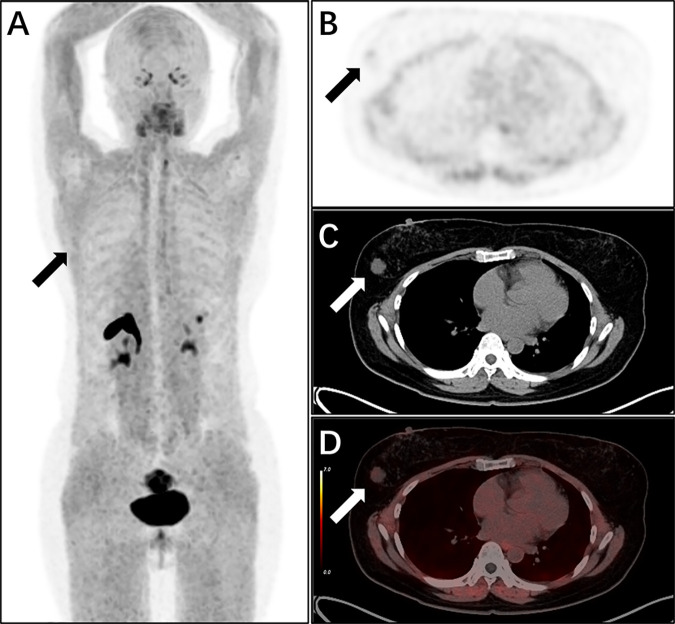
Al¹⁸F-NOTA-FAPI-04 PET/CT images of a 50-year-old female patient with breast mucinous carcinoma (molecular subtype: HER2-positive). (A) Whole-body maximum-intensity projection (MIP) image reveals a mildly FAPI-avid lesion (low to moderate tracer uptake) in the right breast. Solid thick arrows in axial PET (B), CT (C), and fused PET/CT (D) images localize the primary tumor (mucinous carcinoma, a subtype with indolent biology), which exhibits low tracer uptake (consistent with the subtype’s relatively low cancer-associated fibroblast infiltration).
